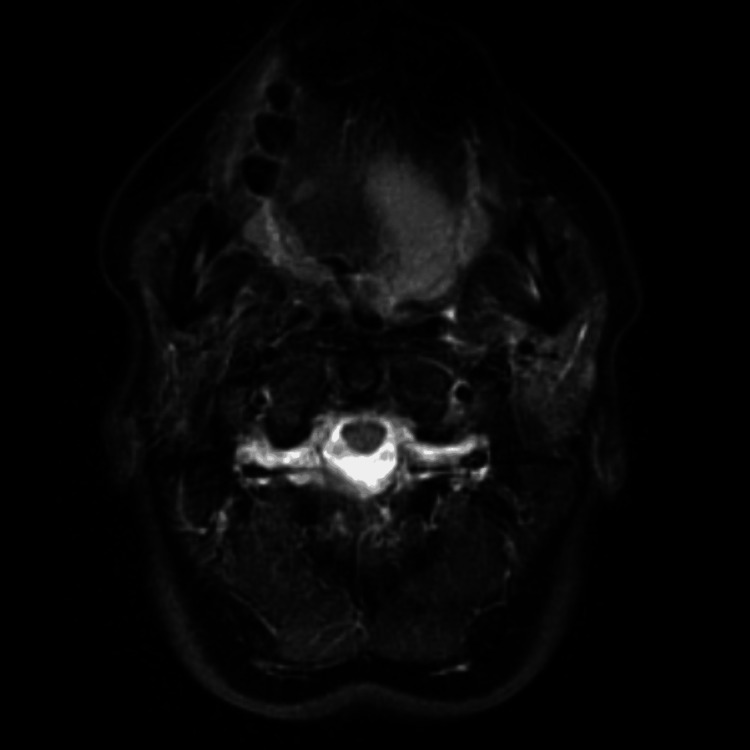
Magnetic resonance imaging short tau inversion recovery axial image showing a mass lesion with relatively uniform high signal intensity on the left lingual side of the root.
